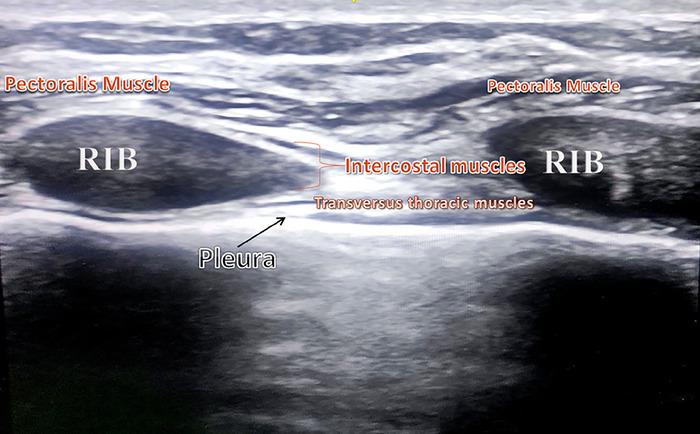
Ultrasound image of anatomic structures with the probe positioned parallel to the sternum at the level of the fourth intercostal space.
